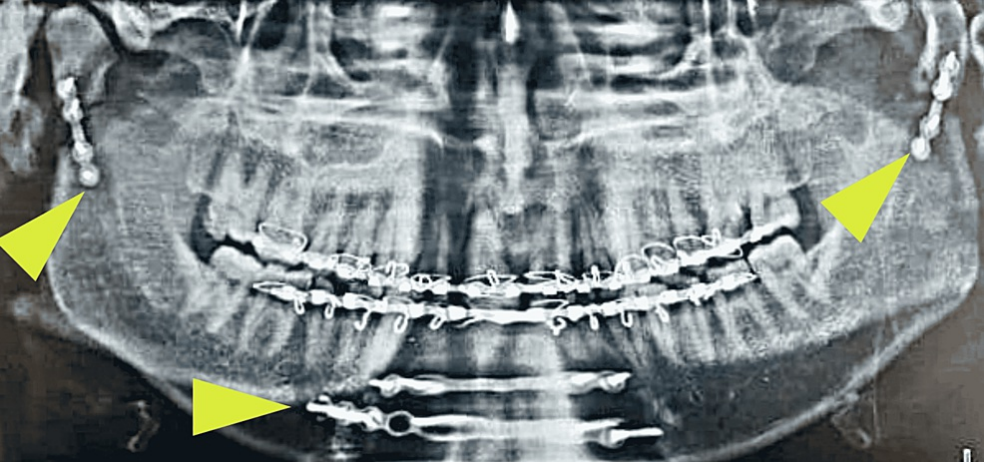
Postoperative orthopantomogram revealing miniplate osteosynthesis at the bilateral mandibular condyles and right parasymphysis region (arrows)
